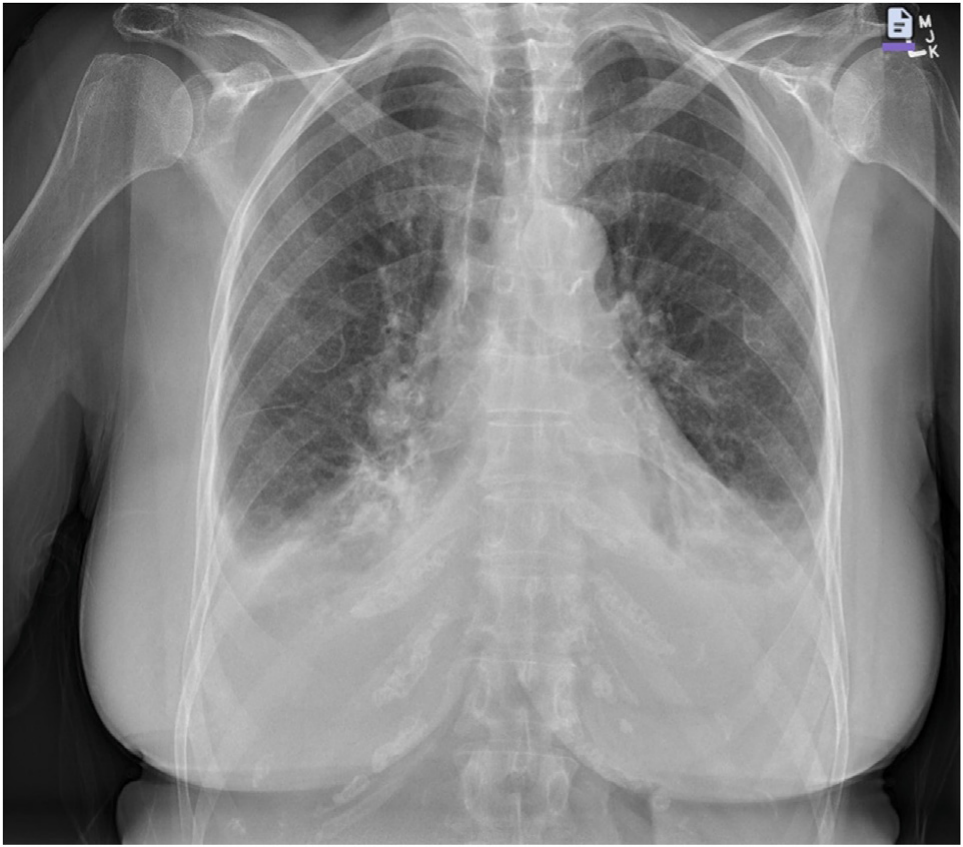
Anteroposterior chest radiography demonstrates pulmonary congestion and bilateral pleural effusions.
